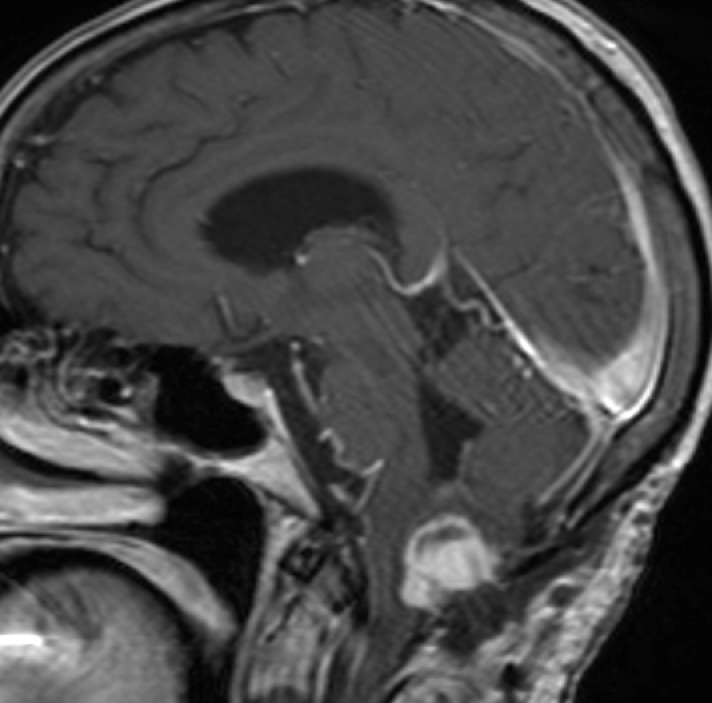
Hemangioblastoma After Attempted Surgical Resection Repeat MRI of the brain after attempted surgical resection showing no significant change in the size of the tumor or associated brainstem compression.
